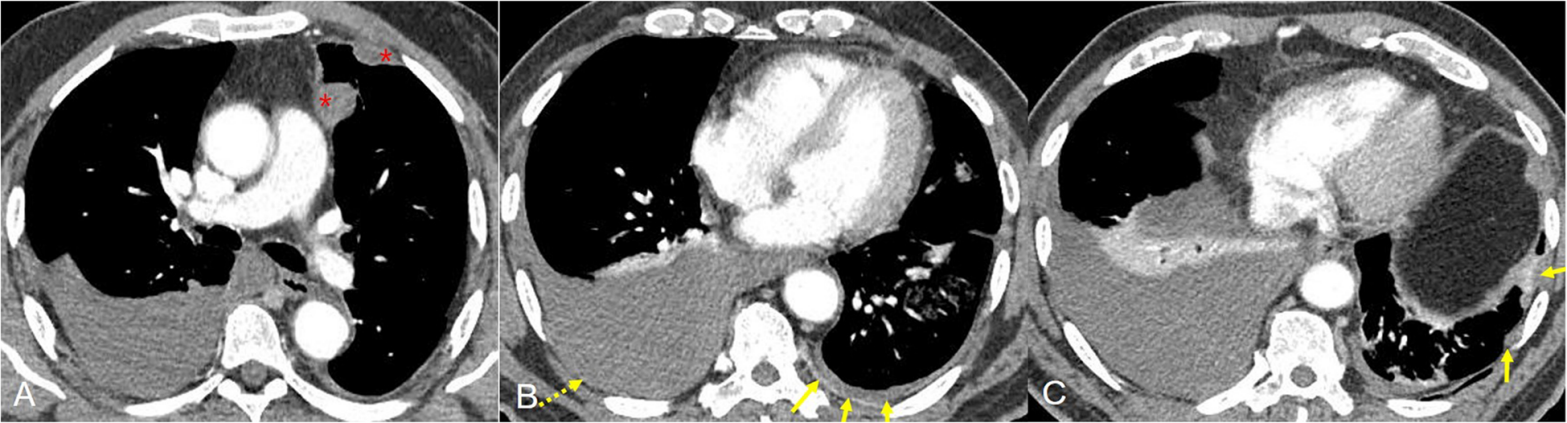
A 65-year-old man diagnosed with primary pleural angiosarcoma. Enhanced, multidetector chest CT scans showed multiple, small nodules of poorly enhancing nature in the left parietal pleura(asterisk) (A). There were also right large pleural effusion and left small pleural effusion with thin, parietal pleural enhancement (B, C)
